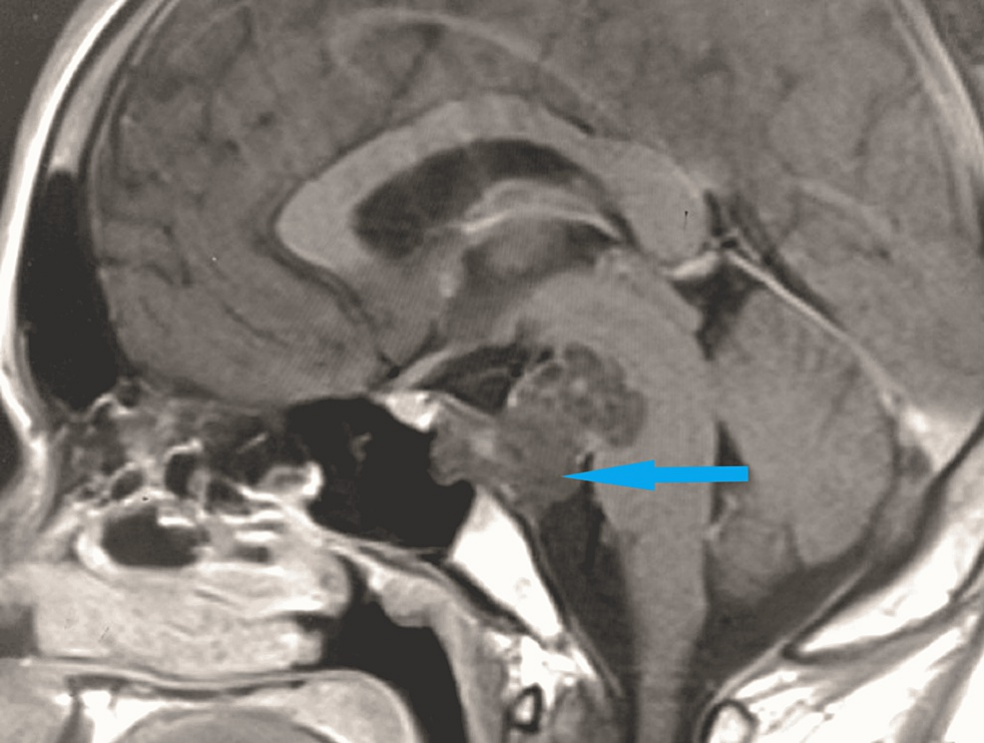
T1 sagittal post-contrast MRI scan of the brain shows no enhancement within the lesion. MRI: magnetic resonance imaging
